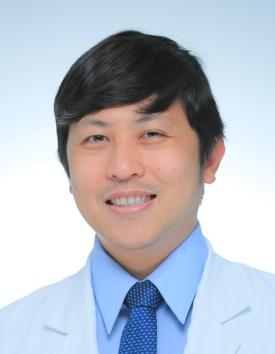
Juan Armando Diaz completed his Medical Degree at the University of the Philippines College of Medicine in 2011 and went on to finish his training in Internal Medicine and Cardiology at the Makati Medical Center, Philippines. He completed his Clinical Research Fellowship in Echocardiography in the same institution before pursuing clinical training in Interventional Cardiology and Transcatheter Aortic Valve replacement at the Sapporo Cardiovascular Clinic, Sapporo, Japan. He is currently a consultant at the Makati Medical Center and heads the Cardiovascular Diagnostics (Heart Station) of the Our Lady of Mount Carmel Medical Center.
